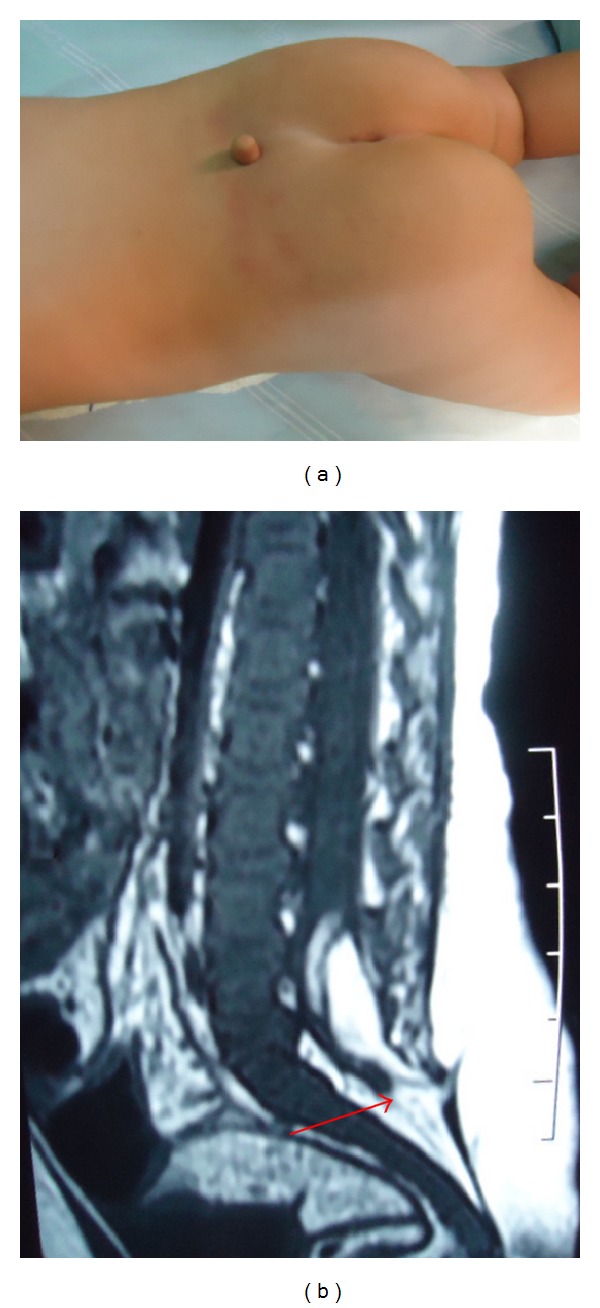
(a) Lesion at the lumbosacral region, type tail. (b) Confirmation by a lumbosacral MRI that showed a spinal dysraphism associated with intradural lipoma.
